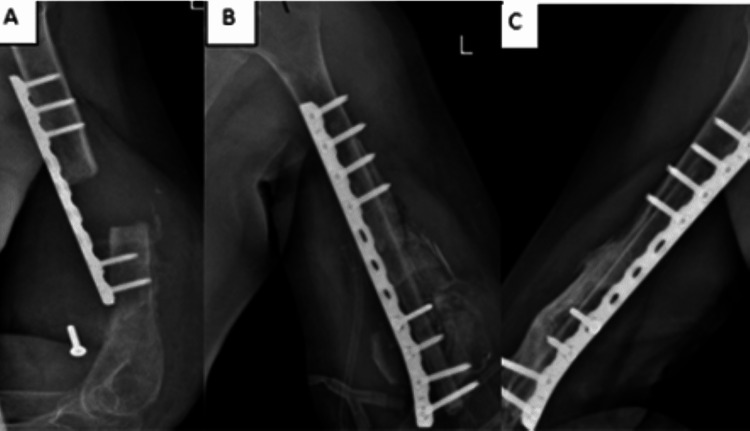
(A) Radiographs showing nonunion with failed osteosynthesis. (B) Immediate postoperative radiograph with LCP and fibular graft. (C) Postoperative image showing healed fracture. LCP: Locking Compression Plating
